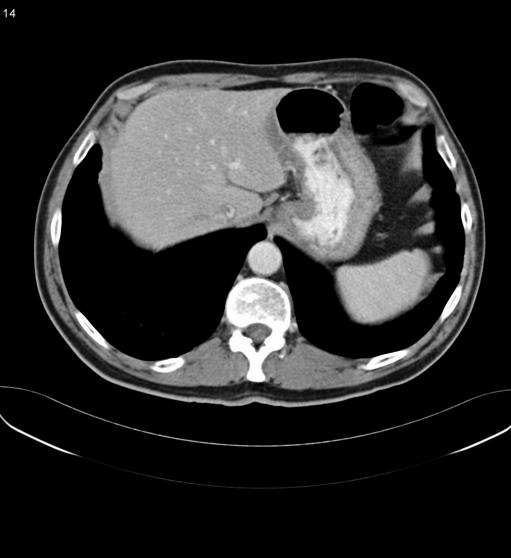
CT at Diagnosis Showing Thickening of Gastro-Oesophageal Junction and Stomach Wall
